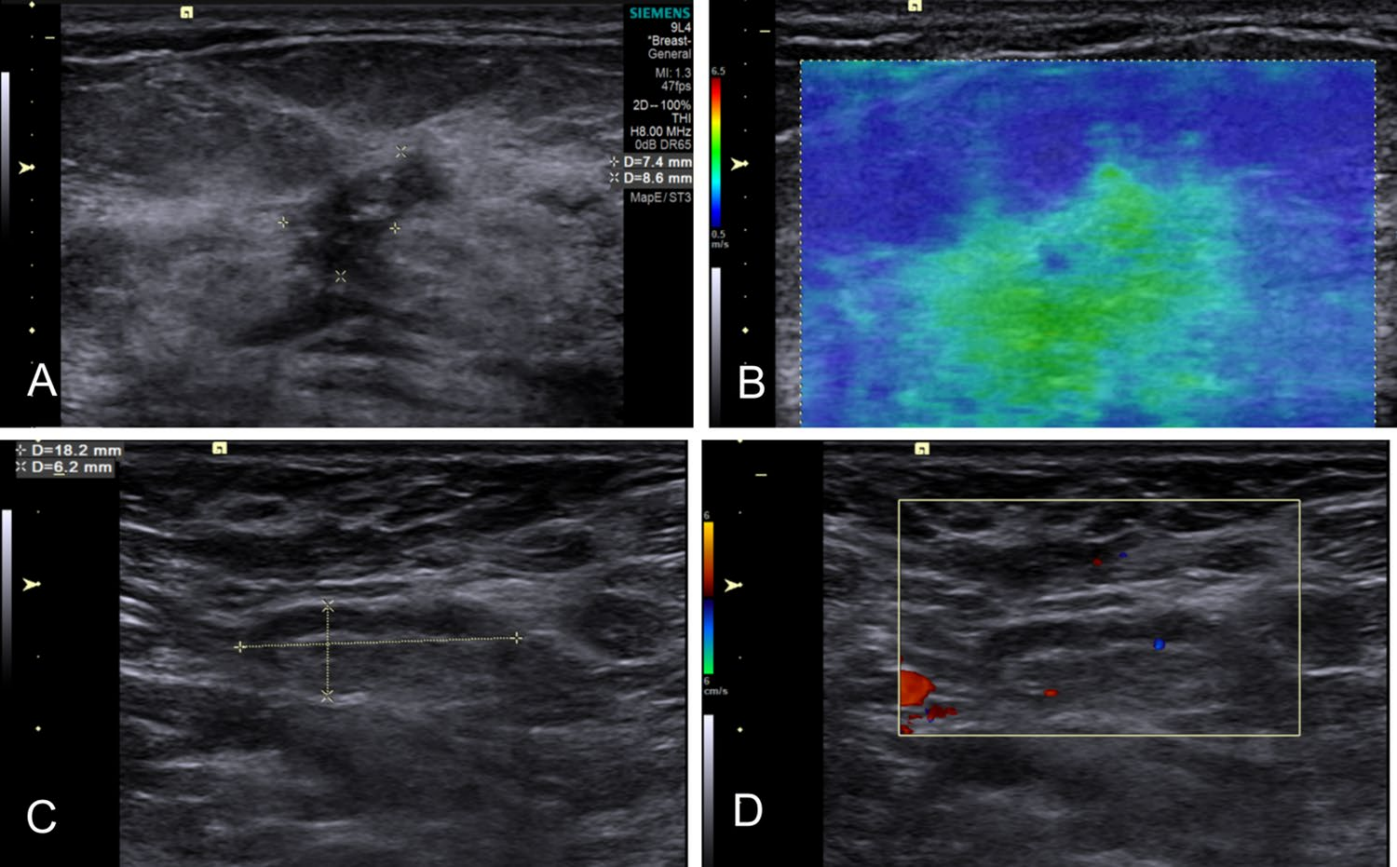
The characteristics of breast SWE and axilla US in a cases with negative axilla level III after NAT. Following NAT, a 50-year-old patient with T2N2 Luminal-B2 breast cancer underwent ALND, during which 21 lymph nodes were removed from axilla levels I-III. According to ALND result, only 1 LNs in axilla level II was found to have residual metastasis. A A solid, hypo-echogenic breast lesion with interior calcification measuring 8.6 × 7.4 mm was found on greyscale US. B The breast lesion showed a SWVmax value of 3.17 m/s on the SWE-velocity map. C–D Several LNs without suspicious features were identified in axilla level I, and no LNs were detected in axilla level II or III on US. Based on the model combing breast SWVmax of 3.17 m/s and negative axilla on US, the predictive result indicated the absence of residual metastasis in axilla level III. SWE, shear wave elastography; US, ultrasound; NAT, neoadjuvant therapy; ALND, axillary lymph node dissection; LN, lymph node; SWV, shear wave velocity
